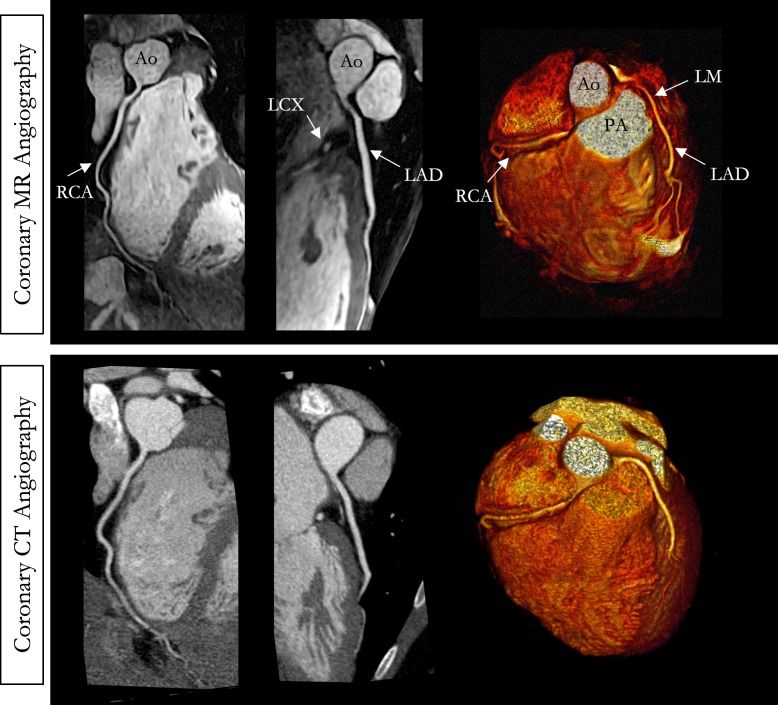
Non-contrast whole-heart sub-millimeter isotropic CMRA images of a 35-year-old male patient with normal coronary arteries (patient 7). Accelerated free-breathing coronary MR angiography (CMRA) images reconstructed with non-rigid motion-compensated PROST are shown in the top row, revealing the LAD and RCA coronary arteries. The 3D CMRA dataset was acquired in 7 min 58 s (heart rate of 57 bpm). The corresponding reformatted images obtained with contrast-enhanced CT coronary angiography are shown in the bottom row. Three-dimensional volume-rendered images for both imaging modalities are shown in the right-hand column. Abbreviations: LAD, left anterior descending artery; RCA, right coronary artery; LCX, left circumflex artery; LM, left main; Ao, aorta; PA, pulmonary artery
